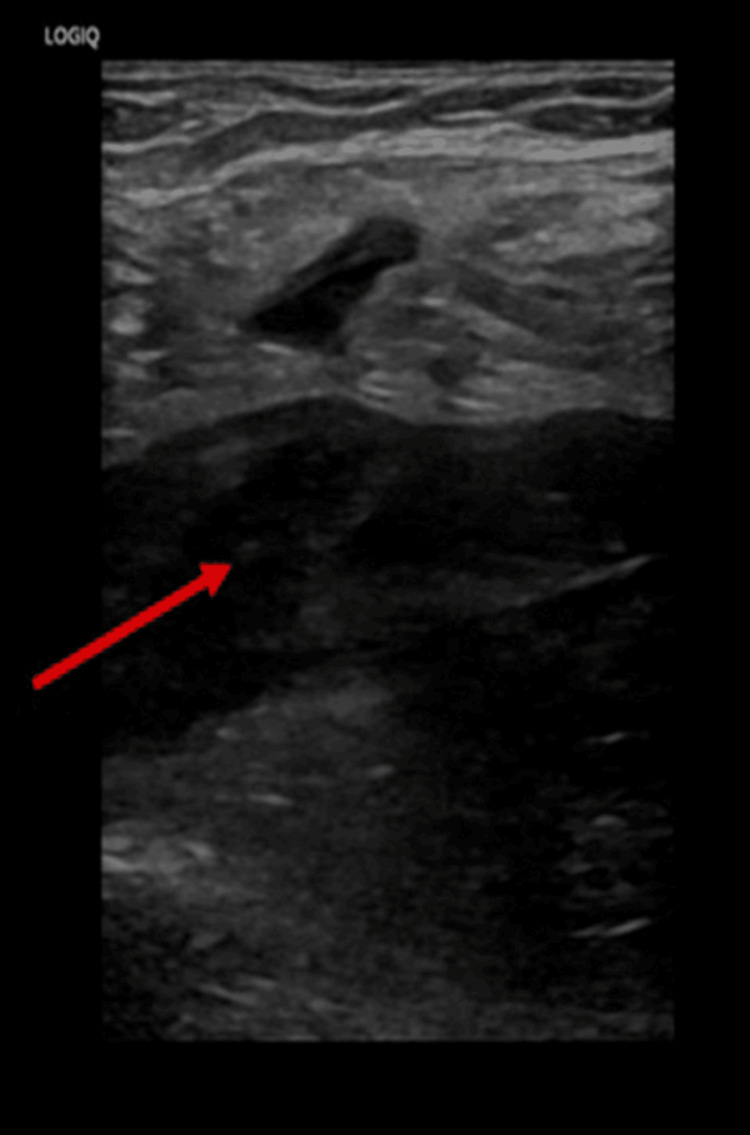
Doppler ultrasound of the left CFV showing a noncompressible vein with intraluminal echogenic thrombus (arrow), consistent with acute deep vein thrombosis CFV: common femoral vein
